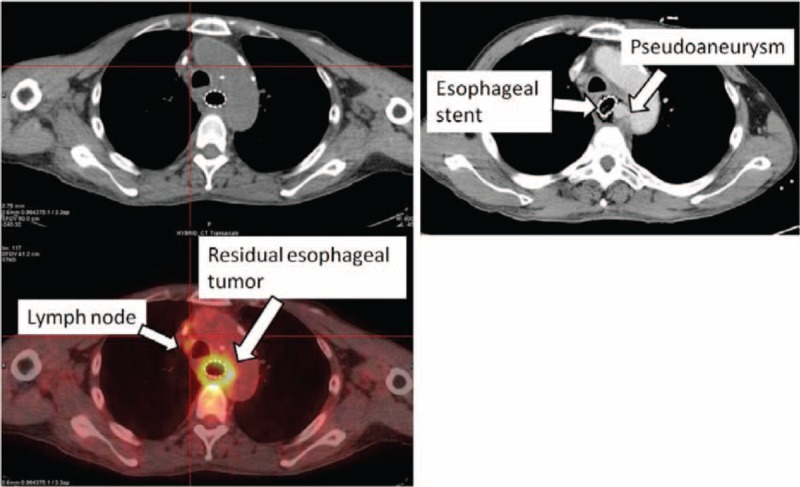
(A) Follow-up positron emission tomography scan 1 month after the completion of concurrent chemoradiotherapy revealed a residual esophageal tumor and enlarged lymph nodes with intact aortic vessel. (B) Chest CT shows a pseudoaneurysm arising from the medial aspect of the proximal descending aorta.
